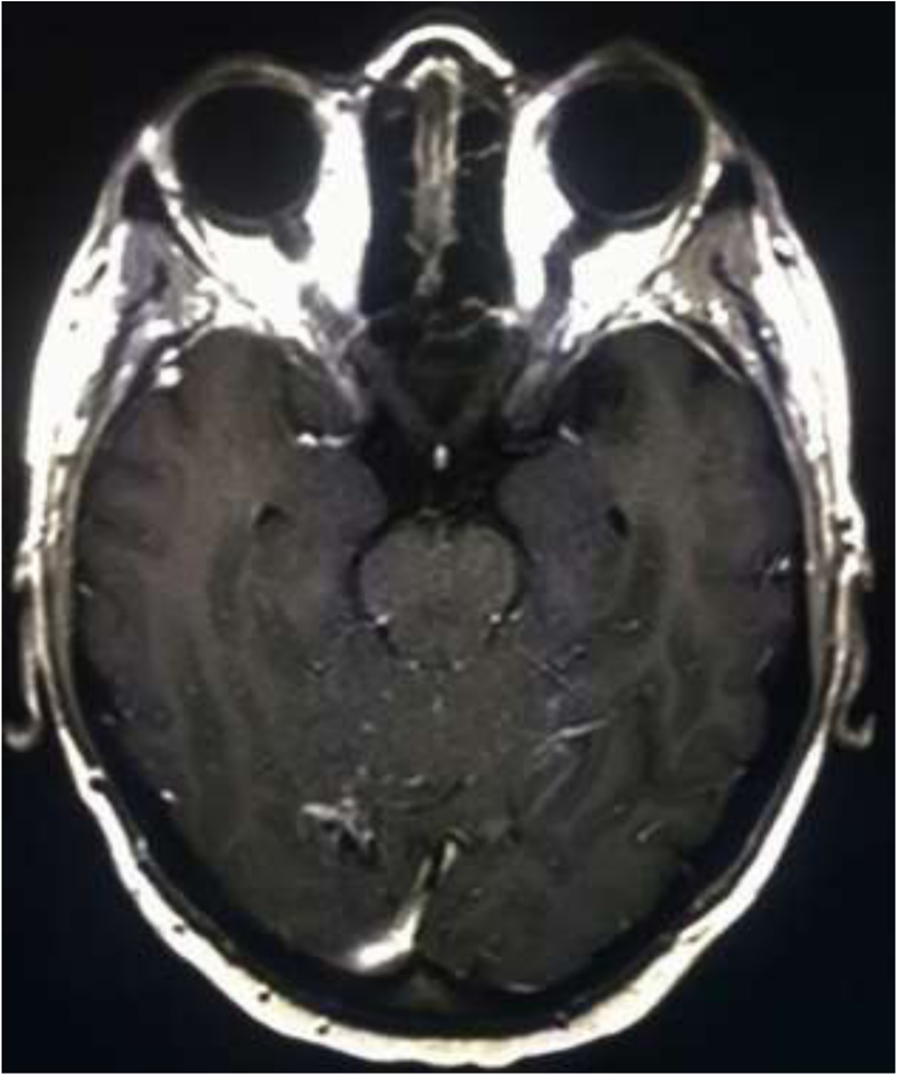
Brain MRI after 3 courses of T-DM1 therapy
